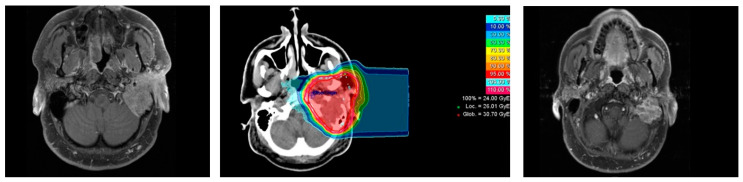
Example of patient with primary acinic cell carcinoma cT4 pN2a cM0; left panel: contrast-enhanced T1-weighted MRI at diagnosis; middle panel: carbon-ion radiotherapy boost plan; right panel: contrast-enhanced T1-weighted MRI at follow-up with in-field local recurrence.
